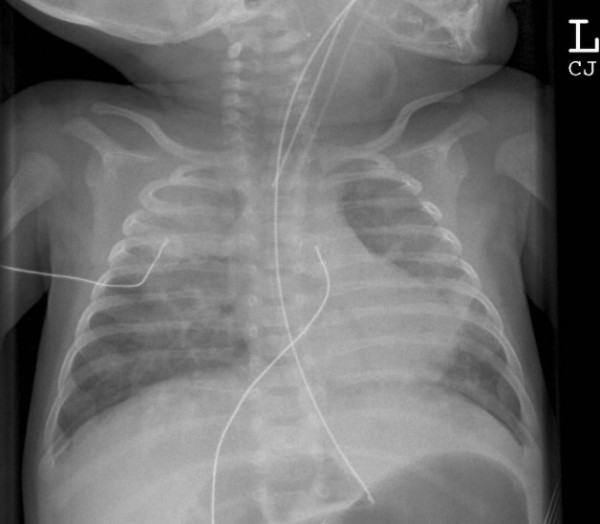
Radiograph taken following episode of aspiration requiring intubation.
